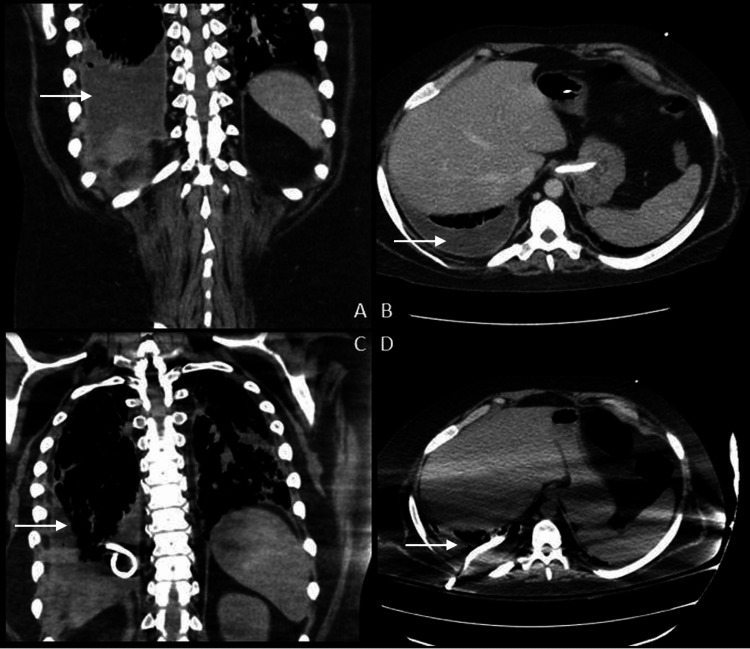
A computed tomography scan of the pleural empyema before and after drainage Figures 1A and 1B were taken prior to the insertion of the pigtail catheter. Figures 1C and 1D were taken after six days of treatment with tPA/DNase. Arrows point to the location of the empyema.
